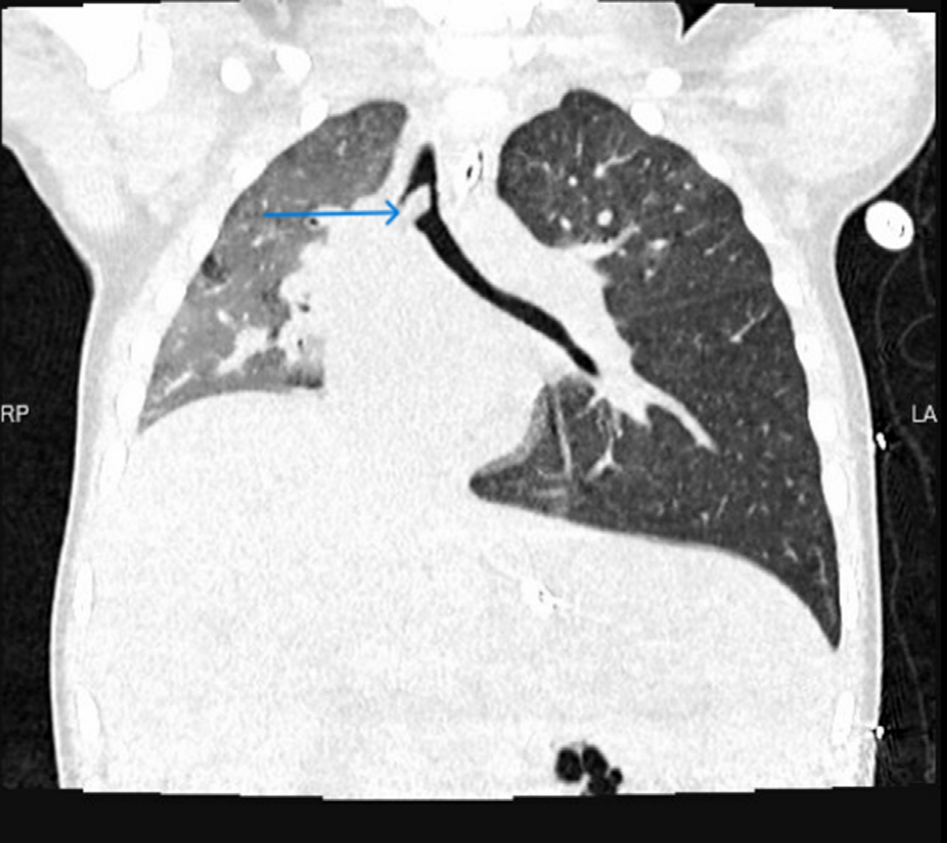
Chest CT scan (coronal view) shows foreign body at level of carina extending to right bronchus (blue arrow). CT, computed tomography.
